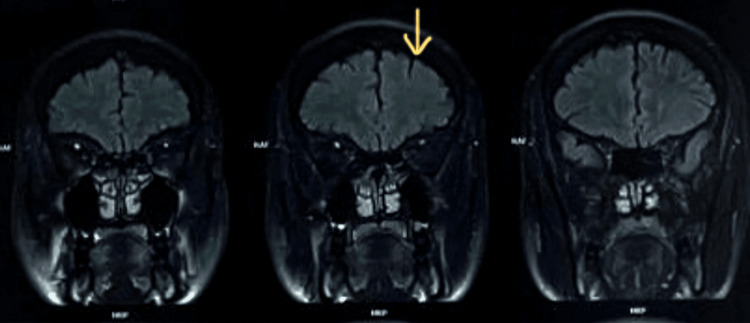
Subacute MRI demonstrating cerebellar cortical laminar necrosis. Coronal/axial T1-weighted images showing curvilinear hyperintensity along the cortical surface of the cerebellar hemispheres, more prominent on the right, in keeping with cortical laminar necrosis. MRI: magnetic resonance imaging
